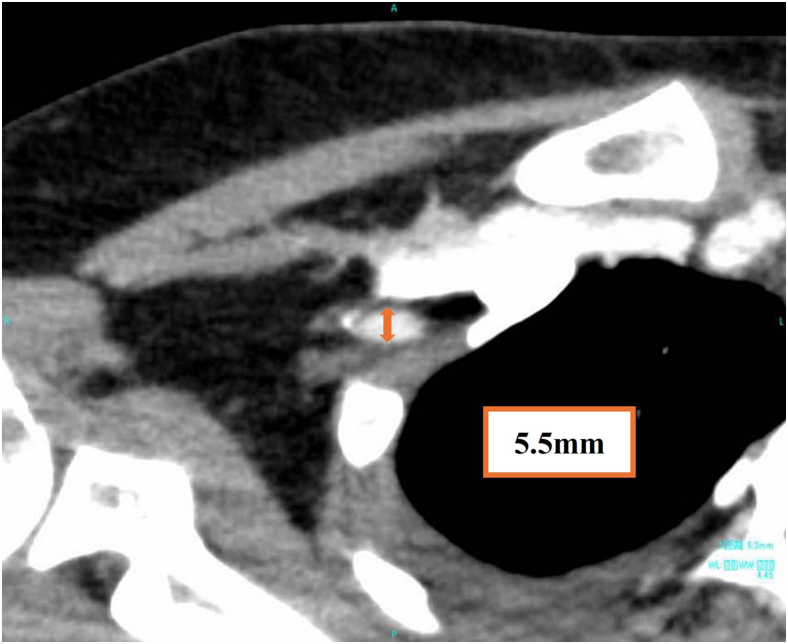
Computed tomography image of the right *AxA*. The minimal artery diameter was 5.5 mm at the first portion of the right *AxA*.
